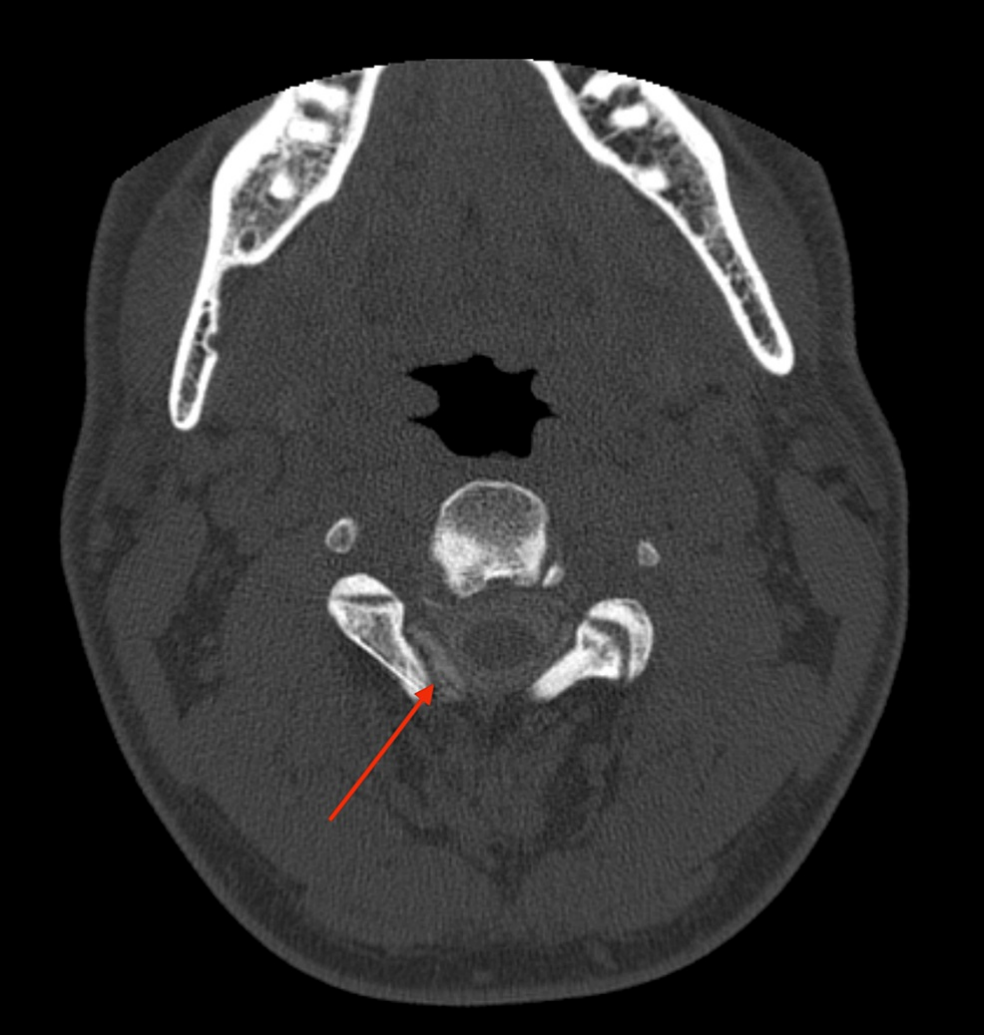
Axial C2-C3 level myelo-CT showing contrast outside the subarachnoid space (red arrow), as evidence of a right-sided C2-C3 CSF fistula.
